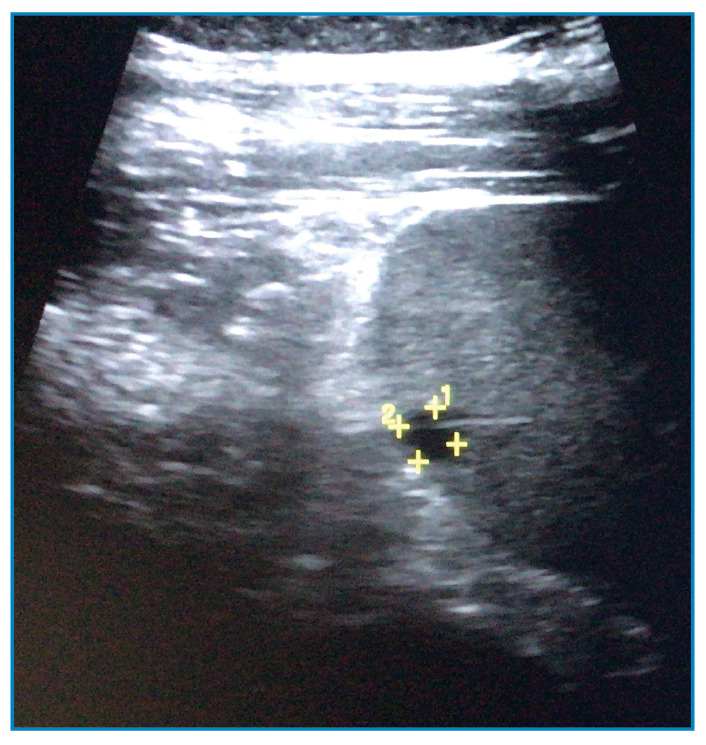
Abdominal ultrasound scan showing a hypoechoic splenic lesion measuring 0.8 cm × 0.8 cm, indicative of an abscess.
